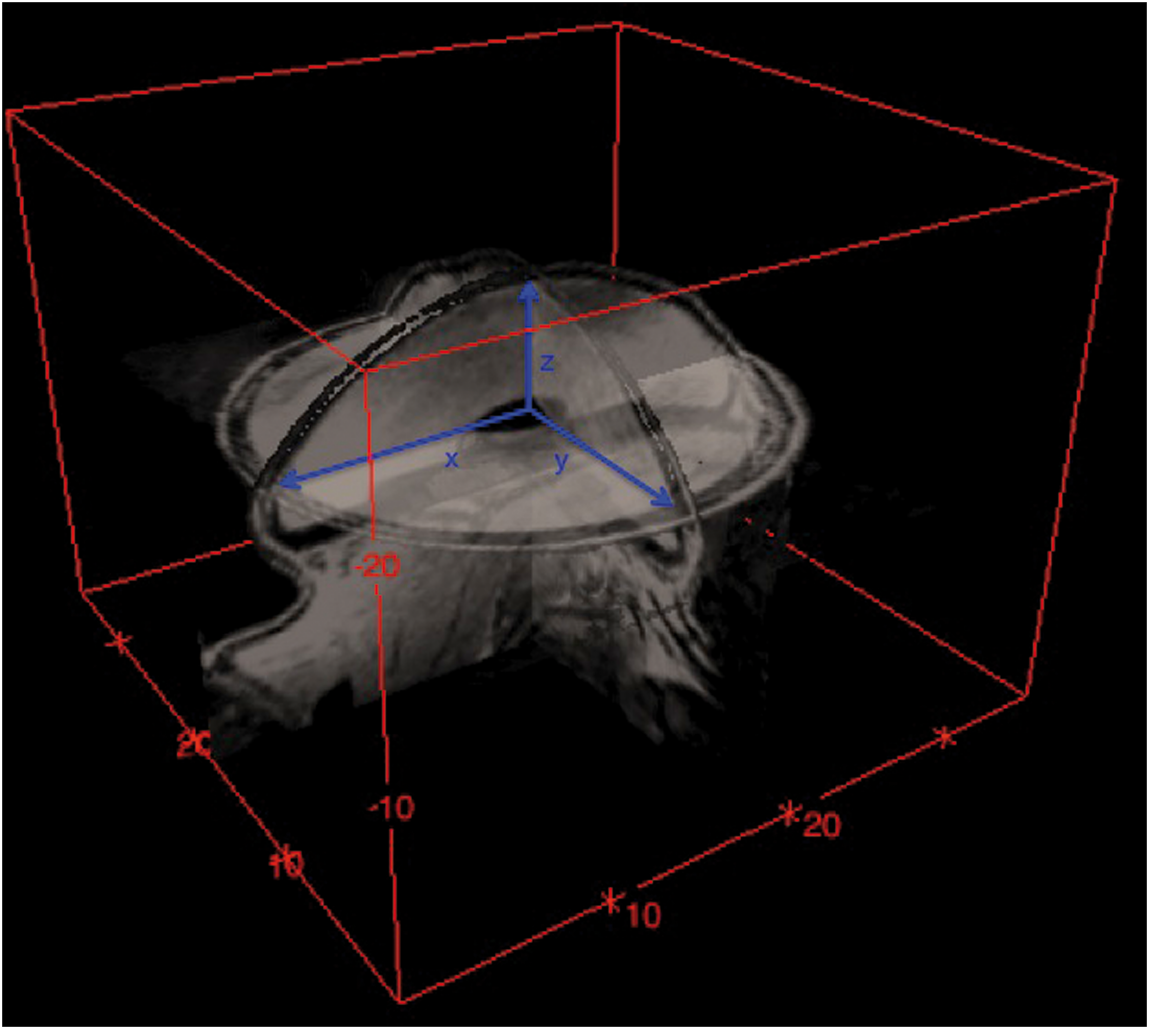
Example picture of MR image after 3D reconstruction and displayed as multi-orthoslice. Measurements were performed at the x-, y- and z-axis (blue arrows). Scale was adjusted automatically by the software.
